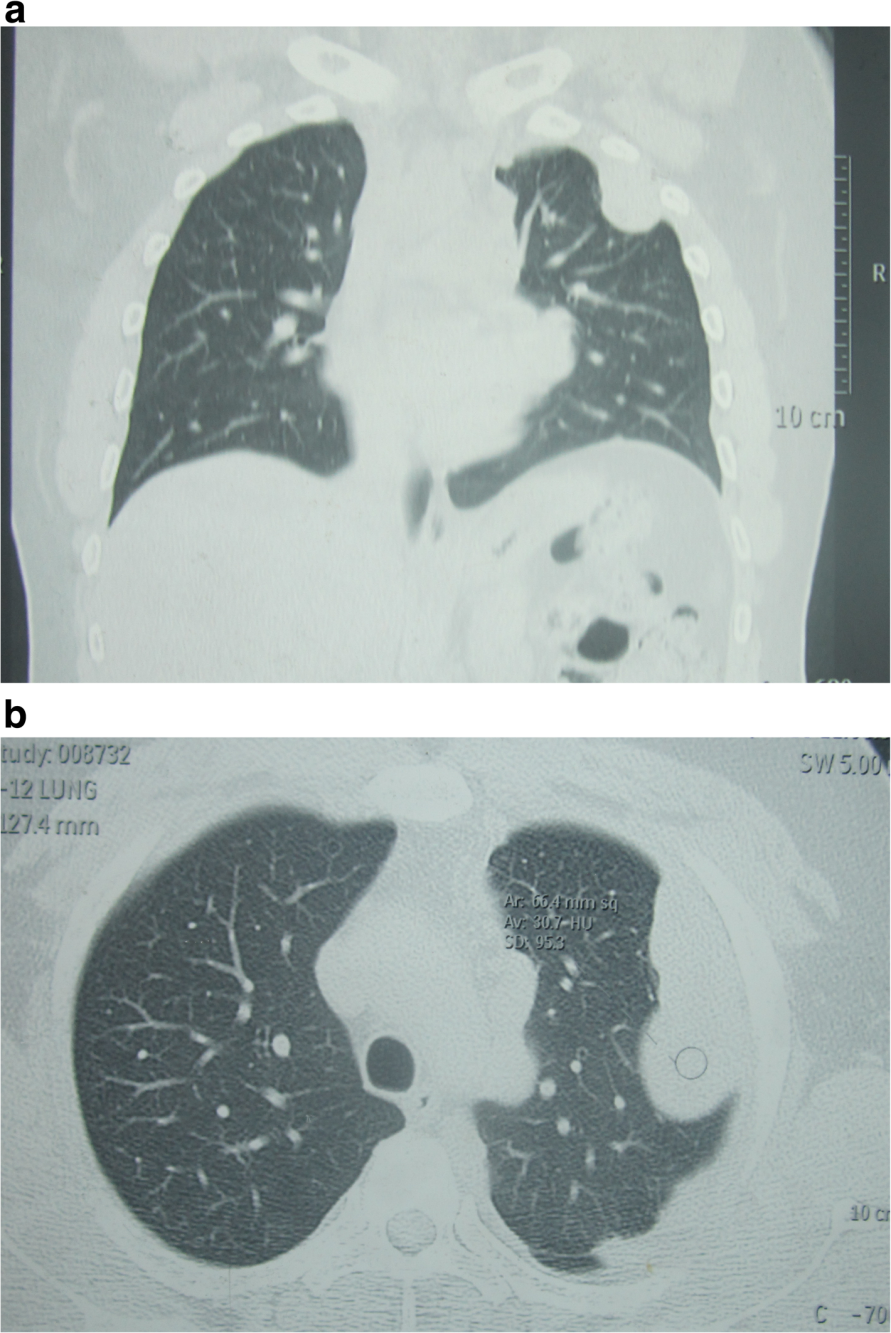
Contrast-enhanced computed tomography of the chest showing mediastinal enlargement and pleural-based deposits. a Coronal view. b Axial view
